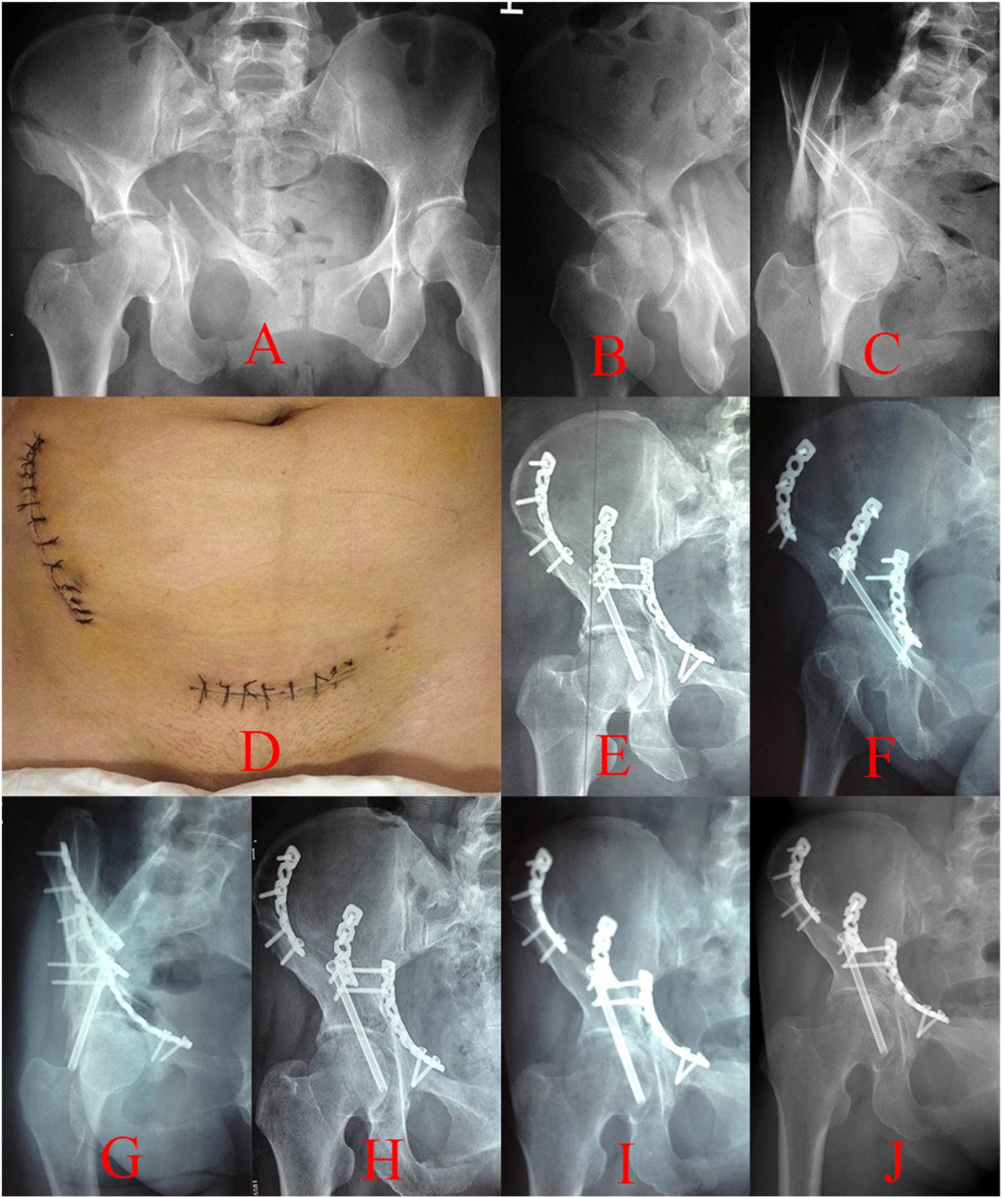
A patient treated with the S+IF approach. (A–C) Preoperative radiographs of pelvis, (D) surgical incision of the S+IF approach, (E) postoperative anteroposterior projection, (F) iliac oblique projection, (G) obturator oblique projection. (H–J) anteroposterior projections in the first, third and sixth months’ follow-up.
